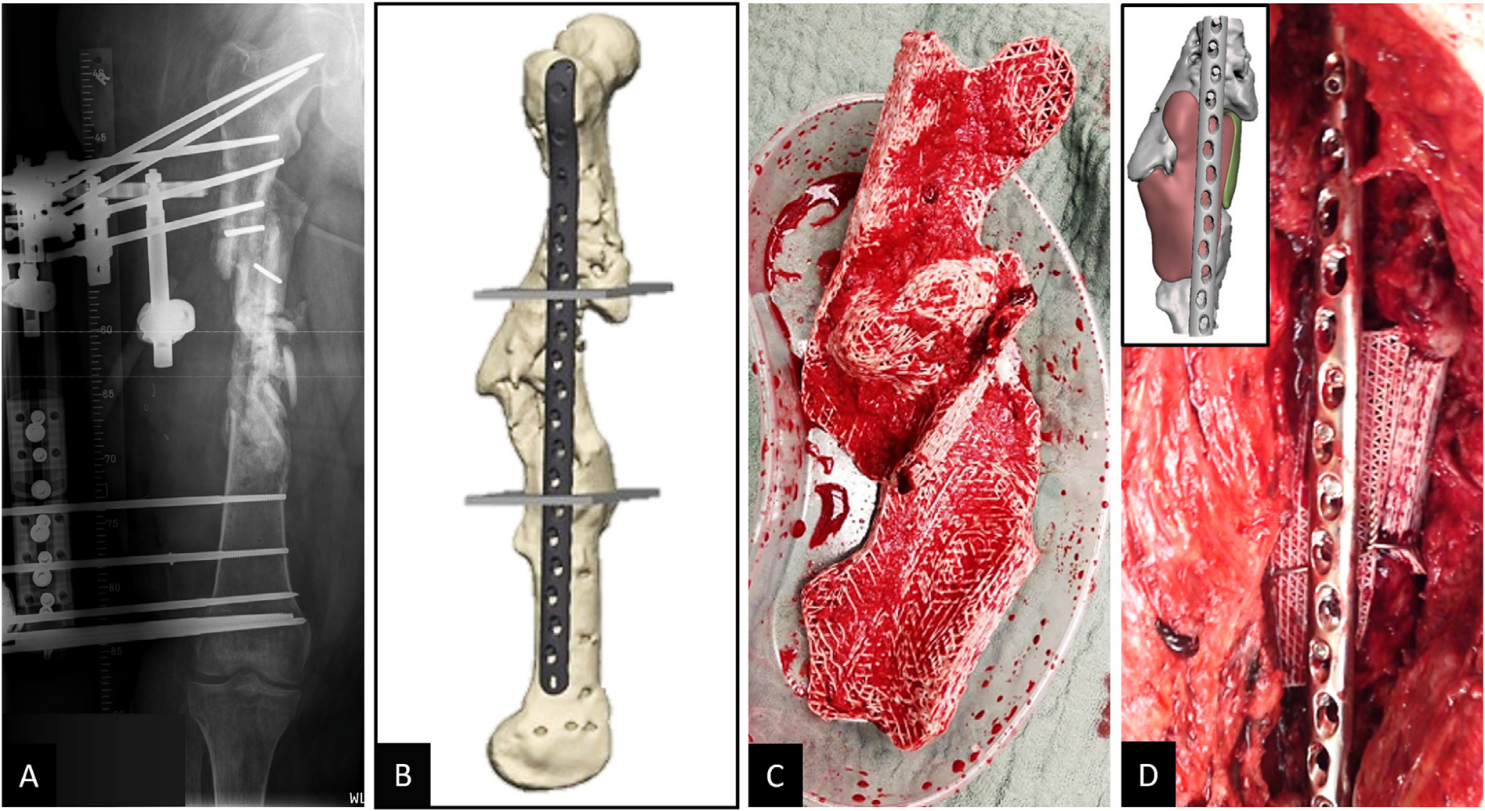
Treatment of large bone defect with complex malunion of the right femoral shaft with modular (two parts) 3D-printed mPCL-TCP scaffolds. Following comprehensive treatment of a posttraumatic septic defect pseudarthrosis, including implantation of a hybrid fixator (Orthofix®) to support stability during infect consolidation (A), 3D reconstruction of CT imaging data showed two distinguished femoral bone defects located antero-lateral and antero-medial and also the preferred plate osteosynthesis was integrated at an early stage in the surgical planning (B). Two geometrically matched 3D-printed mPCL-TCP scaffolds loaded with ABG (C) were implanted and combined with plate osteosynthesis. Proper fit of the modular two-part scaffold of the bone defect as per the pre-operative planning (see inset in D), was confirmed intra-operatively (D).
